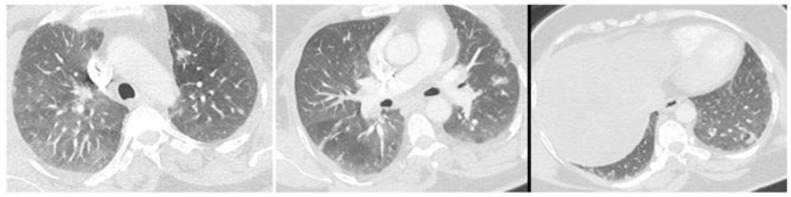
The chest CT scan performed three weeks after the onset of the respiratory sign joint signs, shows some pulmonary condensations.
